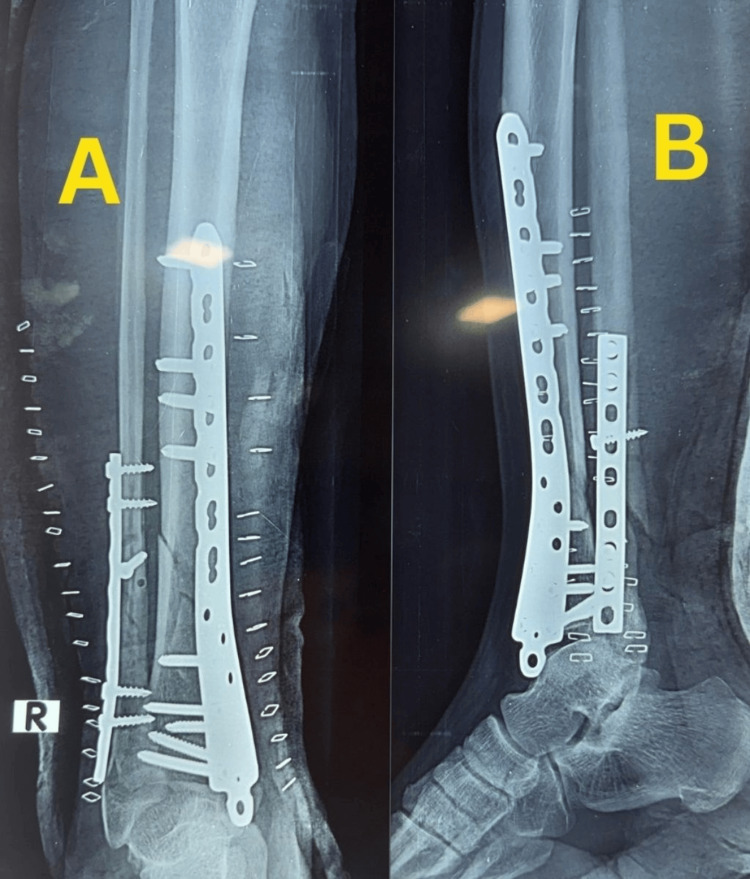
Figure 2. Immediate postoperative radiographs of Case 1. (A) Anteroposterior view showing fixation with a medial distal tibial locking plate and fibular plate with satisfactory alignment. (B) Lateral view confirming stable fixation and restoration of alignment.
